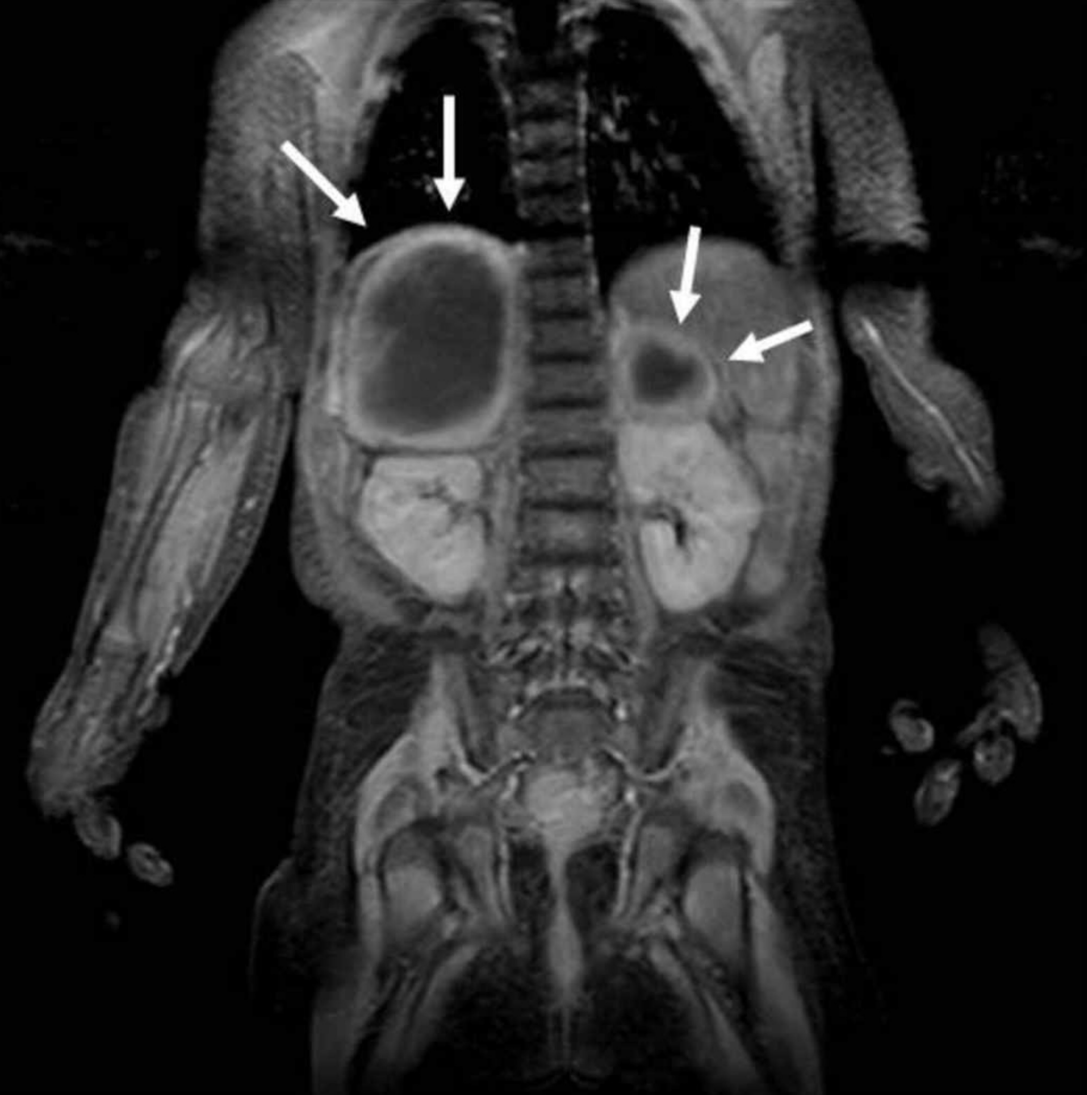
The right adrenal lesion was pushing the right kidney inferiorly. Both lesions had enhancing, thick, irregular walls without solid components on coronal post-contrast fat-saturated T1-weighted image (arrows)
